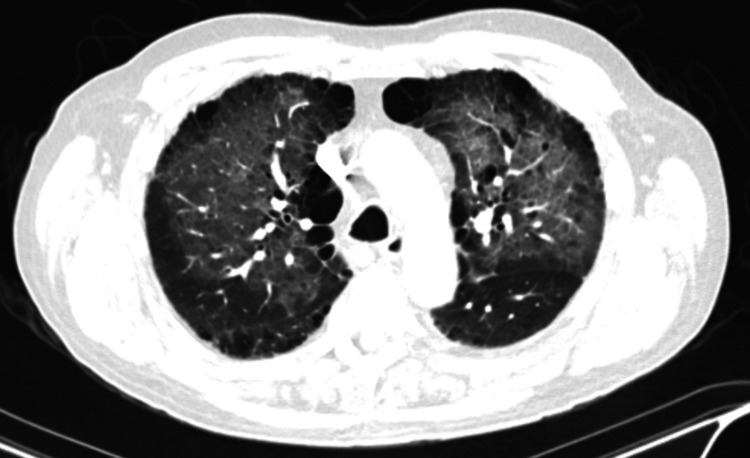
Chest CT scan showing symmetric bilateral ground-glass opacities with associated interstitial thickening within a perihilar distribution most pronounced within the upper lobes in addition to mild para-septal emphysematous changes, and bilateral subpleural reticular opacities suspicious for underlying fibrotic changes
